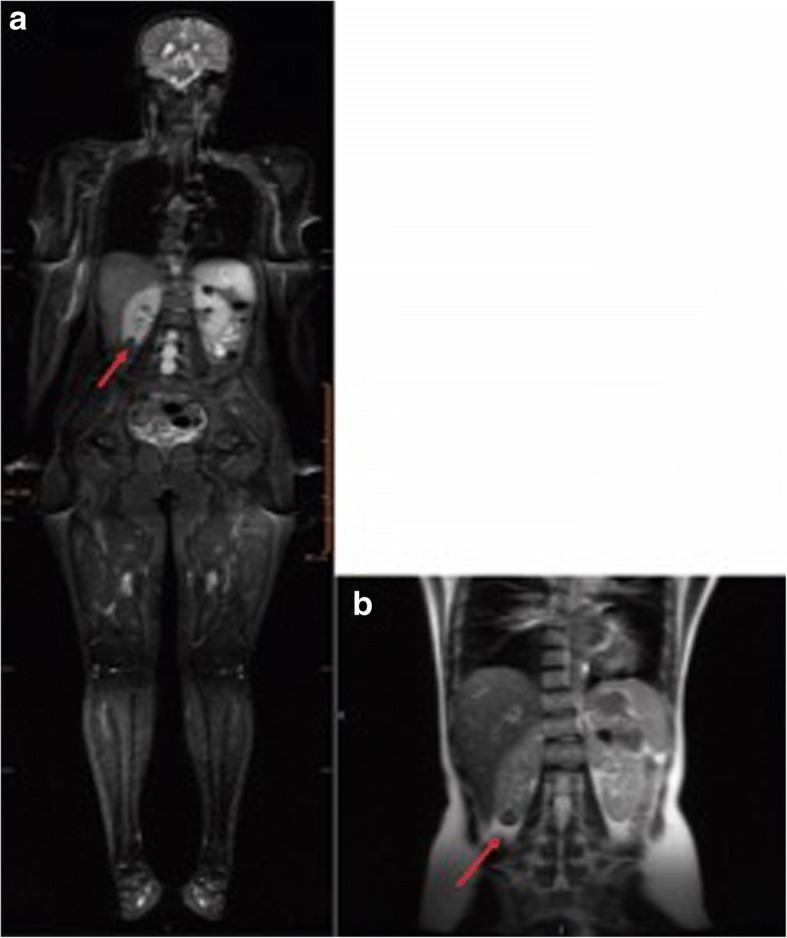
Detection of a renal lesion by WB-MRI in LFS patient, Y0102T000. a STIR image of a 19-year-old with cortical oval kidney lesions in the right lower pole (16 mm) and in the left upper pole (20 mm) (indicated with arrows). b An abdominal MRI detected a complex nodule in the lower third of the right kidney, and it measured 23 × 18 × 18 mm^3^ (indicated with an arrow)
